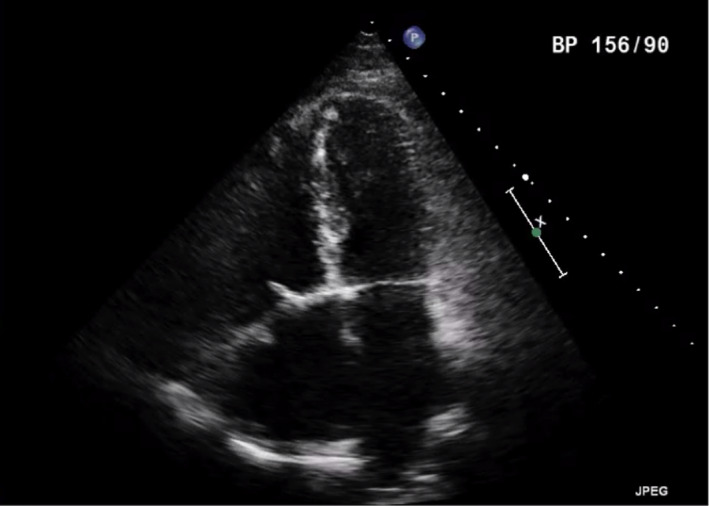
Postoperative Transthoracic Echocardiogram showing TVR
